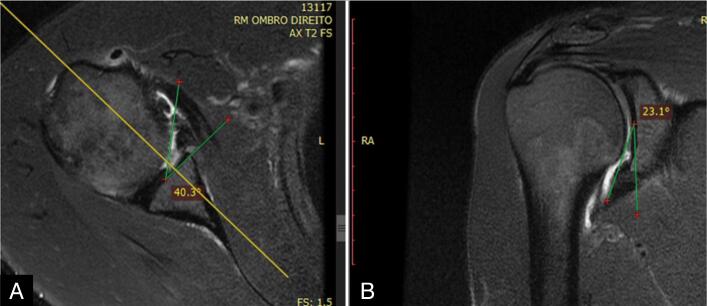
Method for measuring lip angulation, axial section (A) and coronal section (B), on magnetic resonance imaging.
